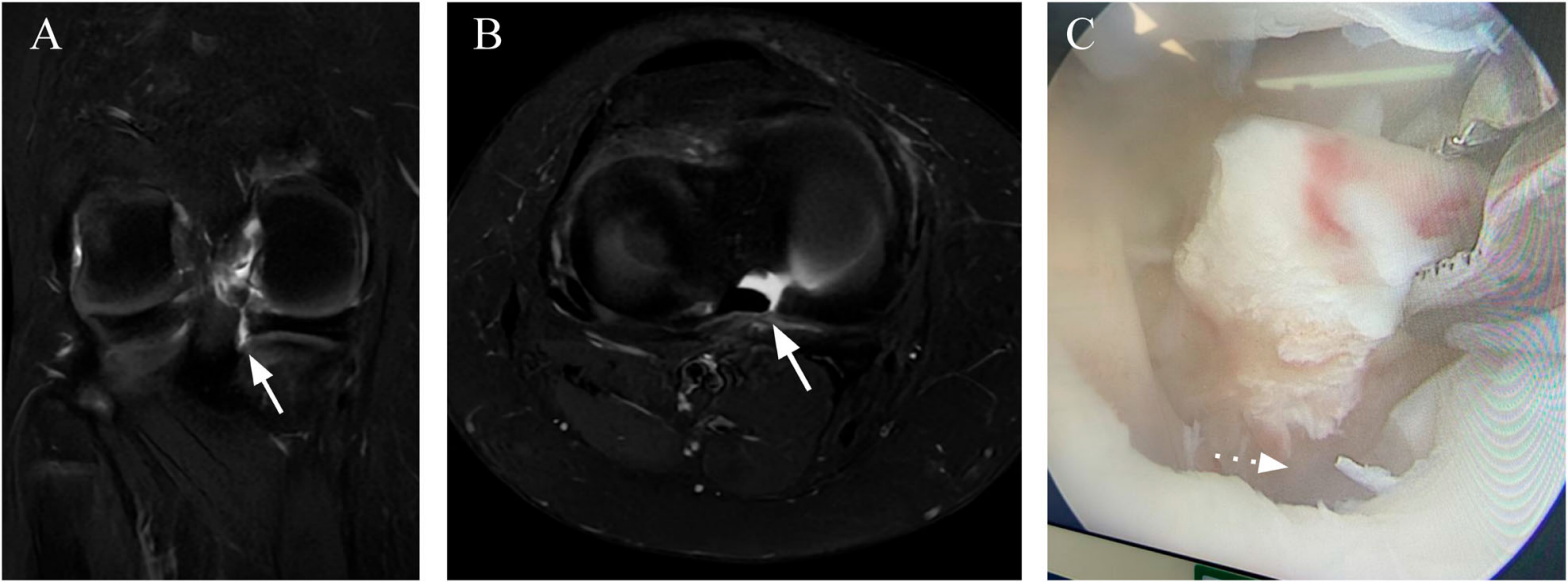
A 9-year-old female with a history of knee pain after falling on roller skates. A Coronal and (B) axial FS T2-W MR images of the right knee showing an MM posterior to the radial root tear (arrow). C Arthroscopy performed a few days later showed a complete radial root tear (dotted arrow)
